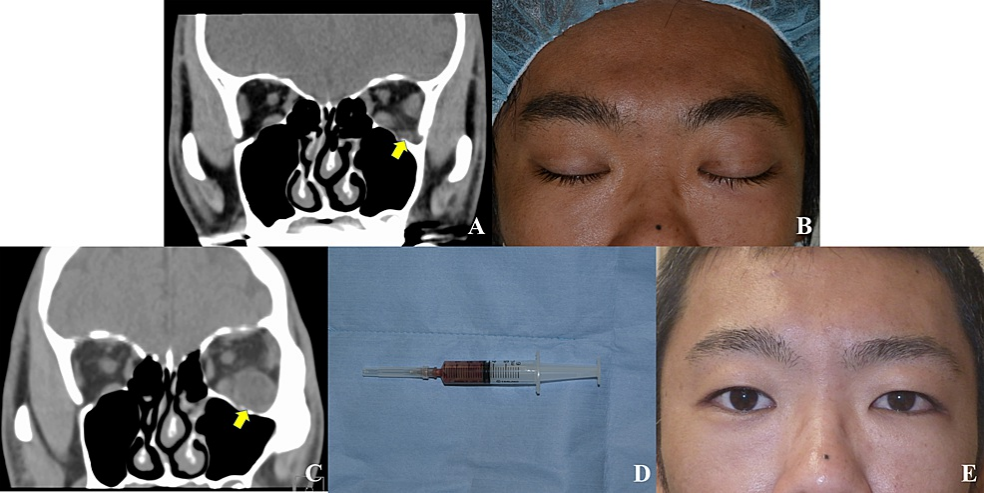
Case presentation. (A) An axial computed tomographic image taken on the first examination showing an orbital mass (arrow) near the inferior orbital fissure. (B) A face photo taken before emergent surgery. The eyelid is swollen on the left side. (C) An axial computed tomographic image taken before emergent surgery showing an enlargement of the orbital mass (arrow). (D) Aspirated orbital abscess. (E) A face photo taken at 1.5 months follow-up showing no swelling in the eyelid and cheek.
